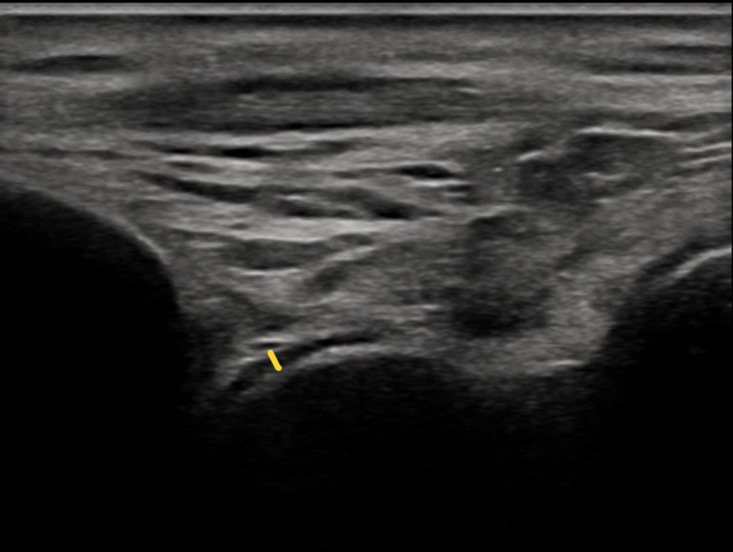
Ultrasonographic measurement of talar cartilage thickness.
